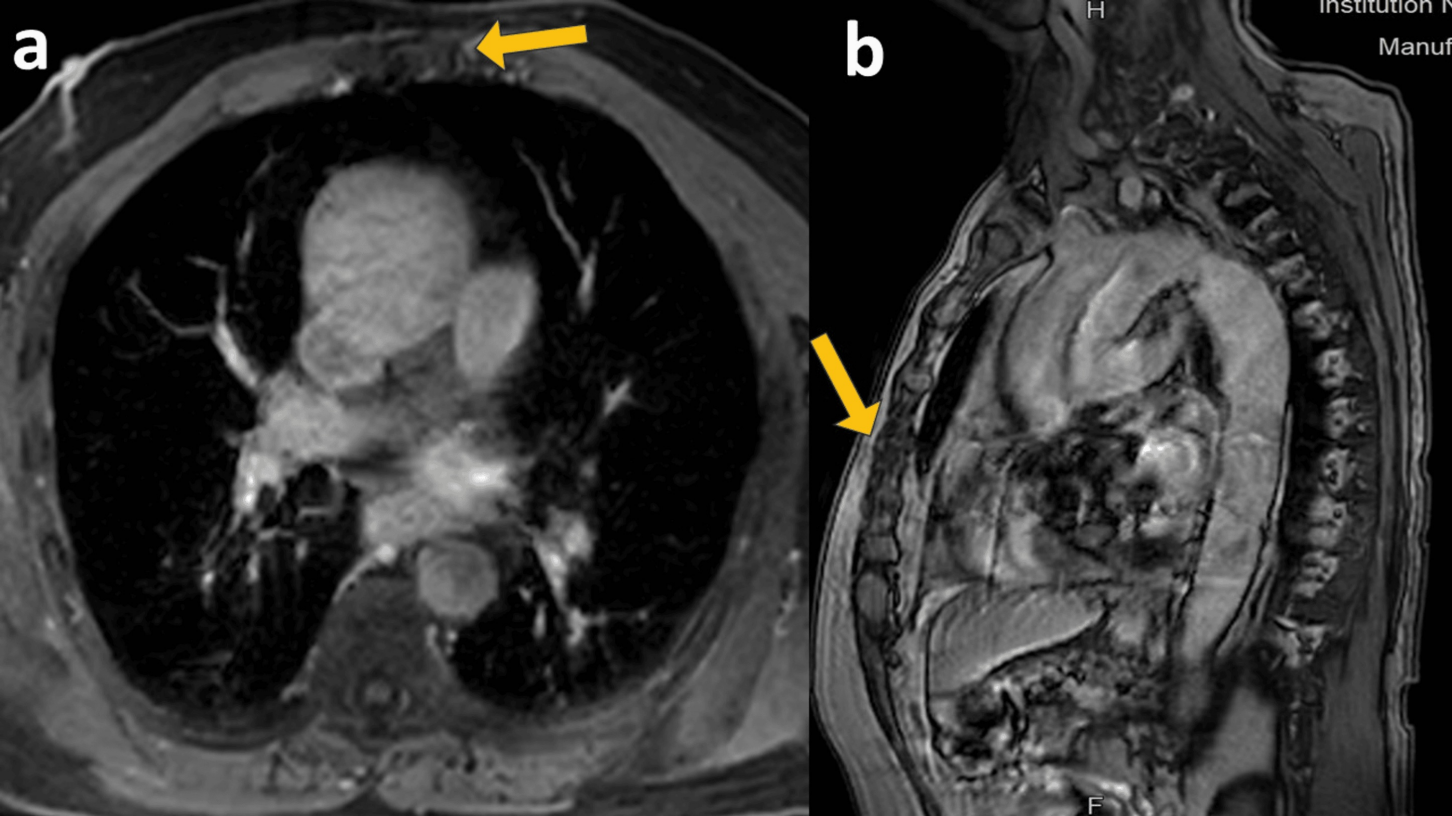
Post-ADT screening MRI of sternal metastatic lesion Screening MRI of the sternal lesion revealed no diffusion restriction, i.e., resolution of sternal metastases. ADT: androgen deprivation therapy
